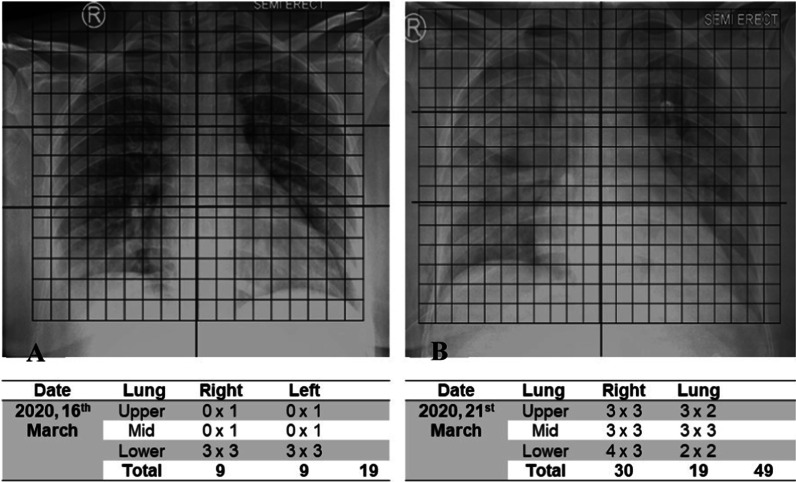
Radiologic severity index assessment in COVID-19 pneumonia patient. This image shows serial chest X-ray RSI values in same patient with COVID-19 pneumonia. A RSI values on initial chest X-rays at 16 March 2020 and B RSI values on serial chest x-rays at 21 March 2020. There is an increase in RSI value at 5-day interval
